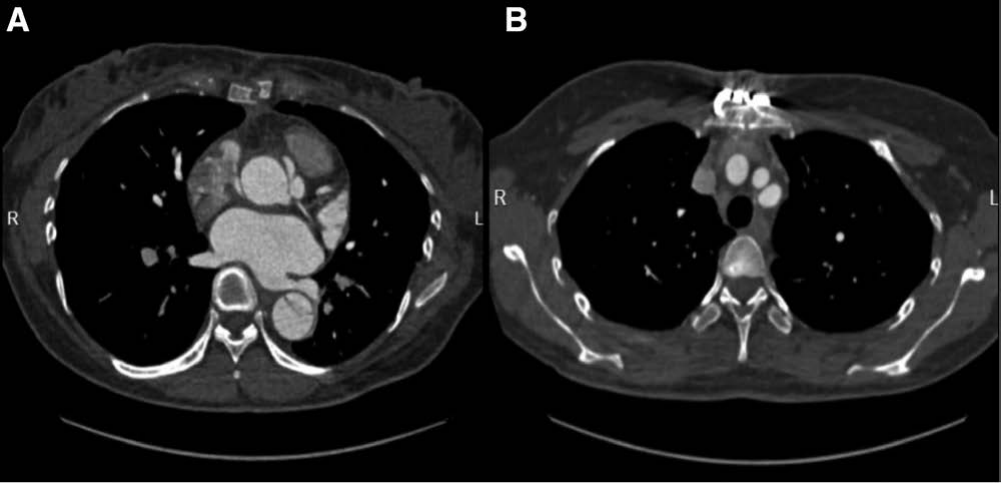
CT examination at 2 months after operation showed that the true lumen of the aorta was enlarged, and no dissection was found in the 3 branches of the aortic arch. CT = computed tomography.
